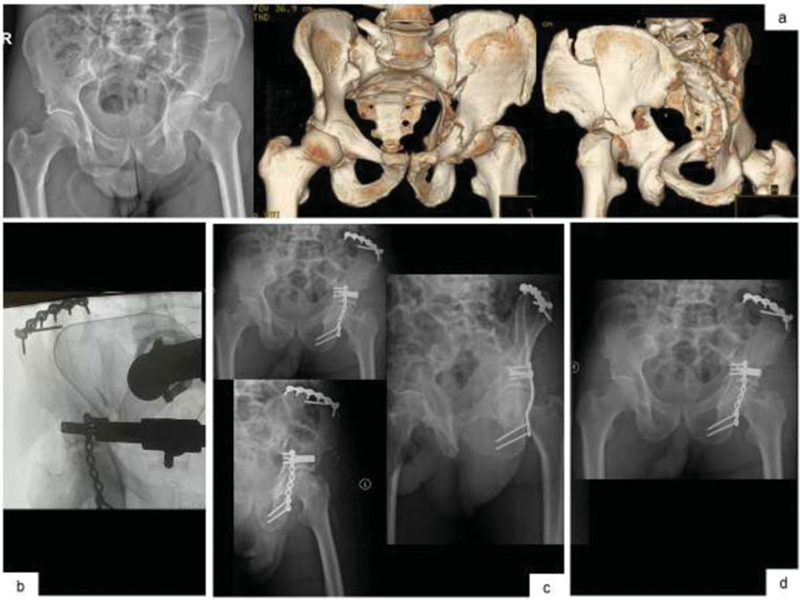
Images of anteroposterior radiography of the pelvis and 3D ACT images for better characterization of the fracture of two columns of the left acetabulum with high extension to the iliac (
